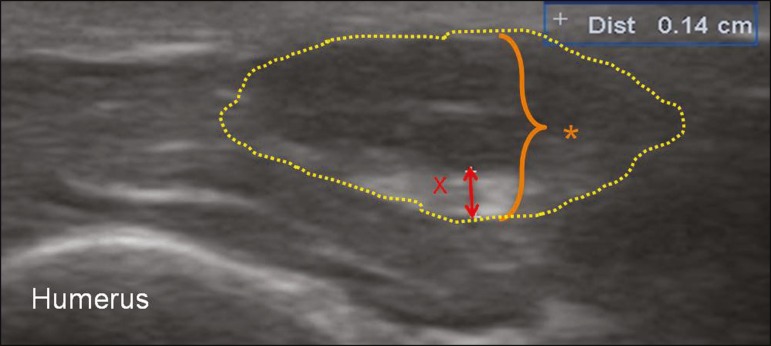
Ultrasound of the left ulnar nerve (asterisk, with contours indicated by
